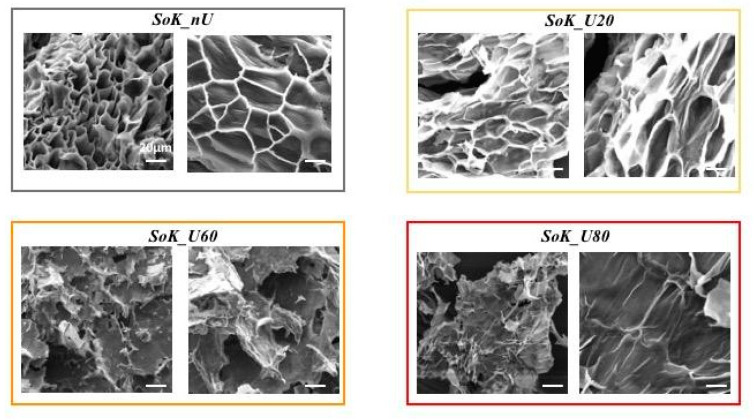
SEM of protein okara obtained with and without the ultrasound treatments. *Scheme 1600*x, r.: 4000x), Sok_U20 (ultrasonicated at 20 °C, magn.: l.: 800x, r.: 1600x), Sok_U60 (ultrasonicated at 60 °C, magn.: l.: 160x, r.: 400x) and Sok_U80 (ultrasonicated at 80 °C, magn.: l.: 400x, r.: 1600x).
